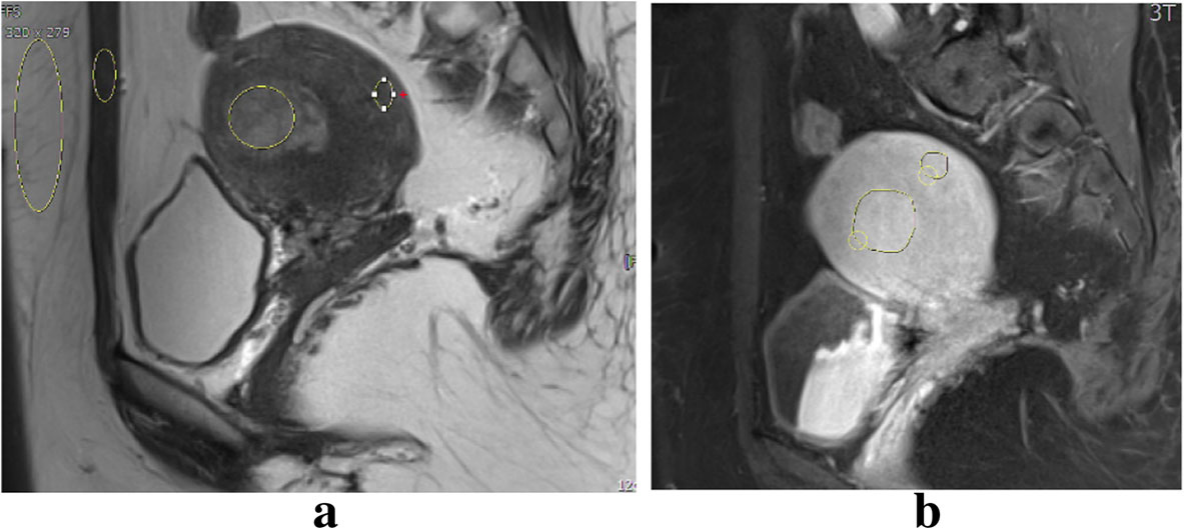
A 34-year-old patient with history of AUB and endometrial stromal sarcoma on pathology. a A round high signal myometrial lesion on sagittal T2-weighted image, tumor myometrial contrast ratio = 0.36, tumor psoas contrast ratio = 1.613. b Sagittal contrast enhanced T1-weighted image with tumor myometrial contrast enhanced ratio = 0.352 and tumor psoas contrast enhanced ratio = 1.482
